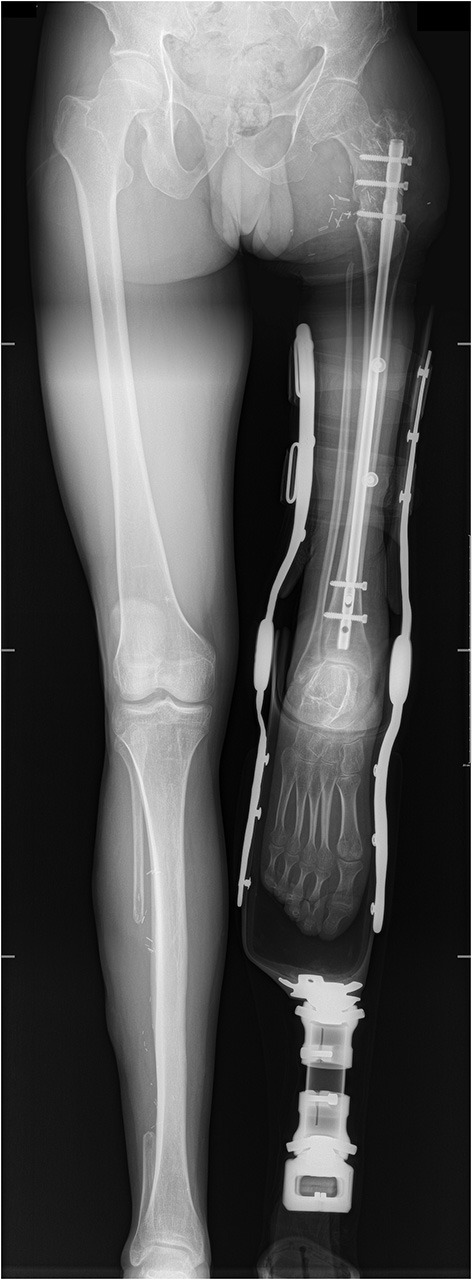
Post-operative X-ray at last follow-up (2 years).
